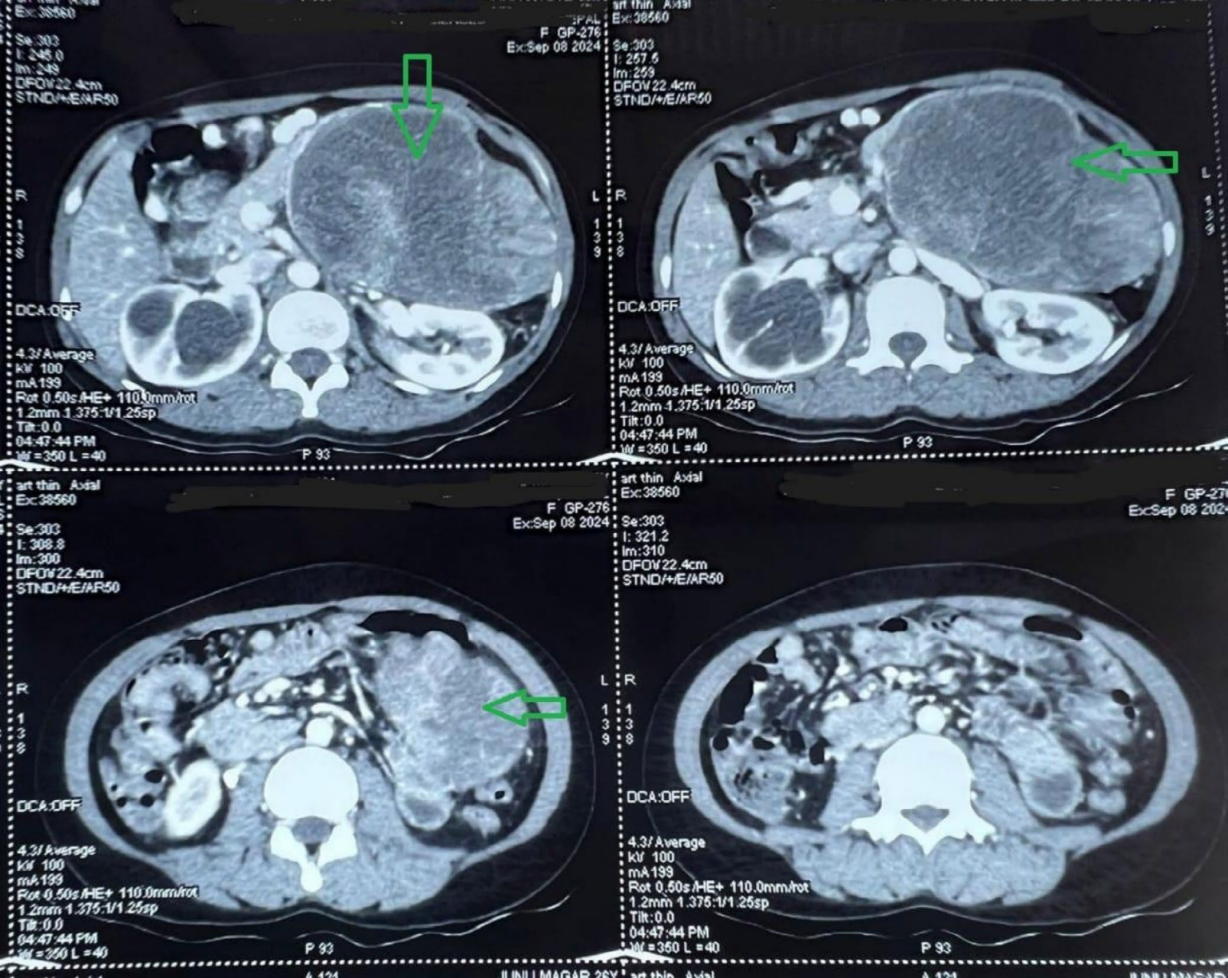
Contrast‐enhanced CT scan of the abdomen showing a large, well‐encapsulated heterogenous mass arising from the body and tail of the pancreas, with solid and cystic components, internal hemorrhage, and invasion into the spleen and splenic flexure of the colon. The right‐sided pelvicalyceal system is also dilated.
